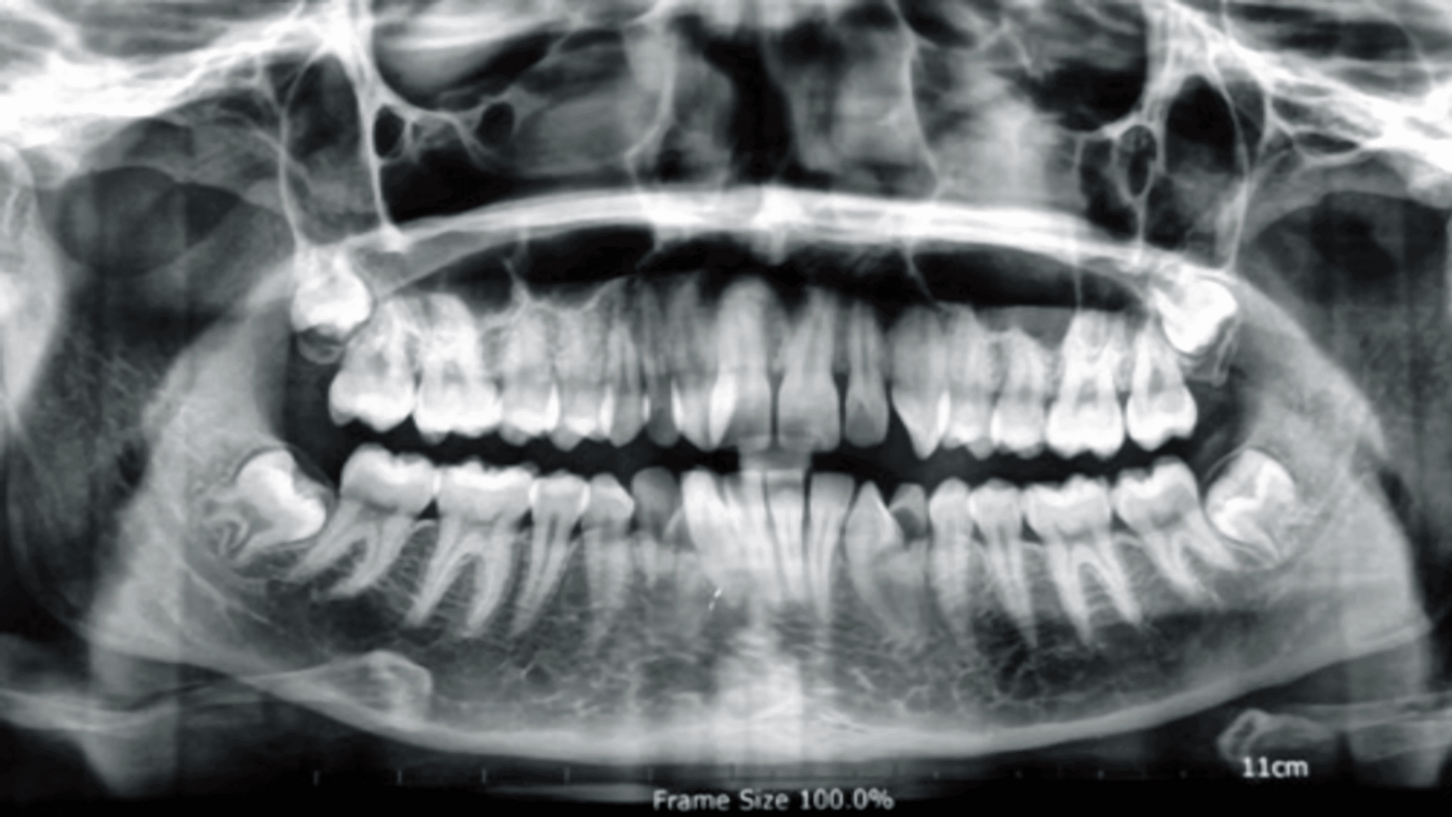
Pretreatment orthopantomogram
